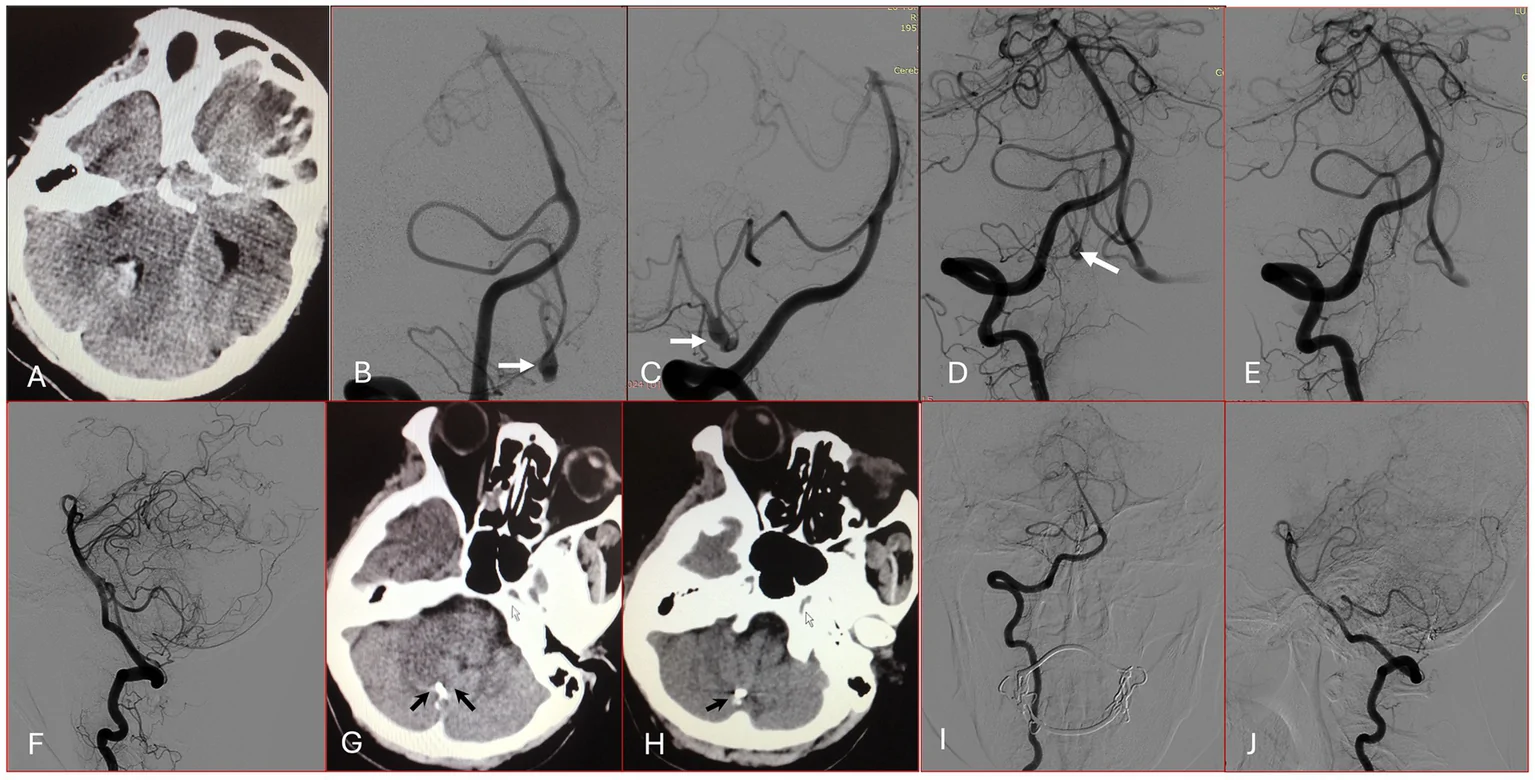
Case 16. 57-year-old female patient presented with sudden-onset headache; head computed tomography (CT) demonstrated subarachnoid hemorrhage (SAH). (A) Head CT demonstrates subarachnoid hemorrhage (SAH) and hemorrhage within the fourth ventricle, accompanied by mild hydrocephalus. (B,C) Right vertebral artery angiograms [anteroposterior (B) and right oblique (C)] show a 7 × 2.5 mm saccular-configured dissecting aneurysm (white arrow) with a daughter sac and a 2.5-mm neck, located at the cortical segment of the posterior inferior cerebellar artery (PICA), with an extremely tortuous vascular access. (D) The tip of the Marathon microcatheter (white arrow) was positioned within the aneurysm sac. (E,F) Right vertebral artery angiograms [anteroposterior (E) and lateral (F)] obtained immediately after parent artery occlusion (PAO) with Onyx-18 show complete occlusion of the aneurysm and the adjacent parent artery, with robust collateral circulation between the proximal and distal branches of the occluded parent artery. (G,H) Head CT obtained on post-procedural day 3 shows no infarction in the PICA territory and Onyx deposition within the lesion (black arrow). (I,J) Follow-up angiograms at 12 months post-procedure [anteroposterior (I) and lateral (J)] demonstrate stable complete aneurysm occlusion.
